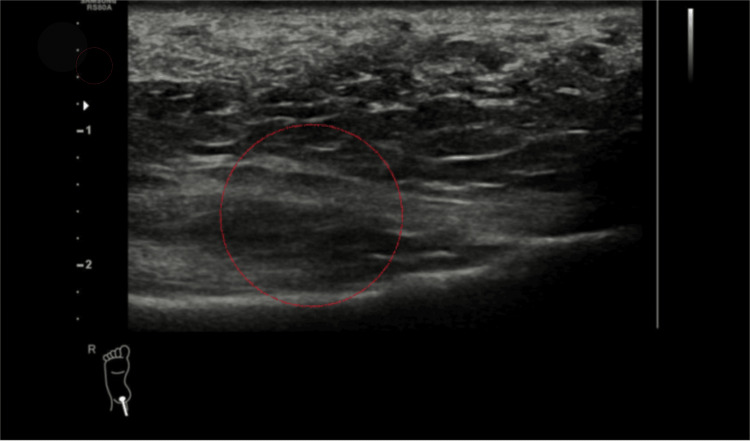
Representative US image showing reduction in the thickness of the fascia at six months after treatment with SVF - case 3 US: ultrasound; SVF: stromal vascular fraction
